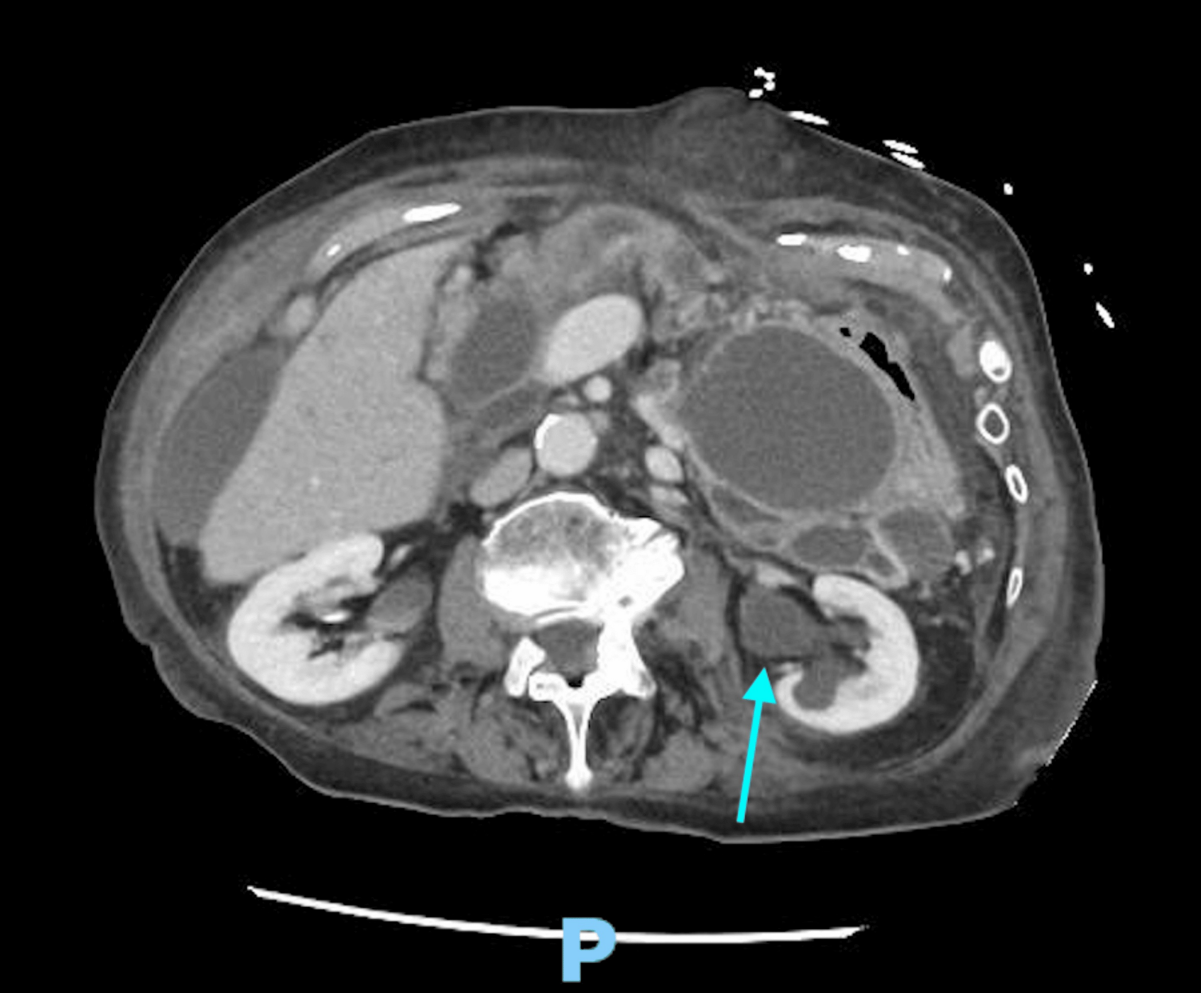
CT abdomen and pelvis with oral and intravenous contrast showing hydronephrosis.
